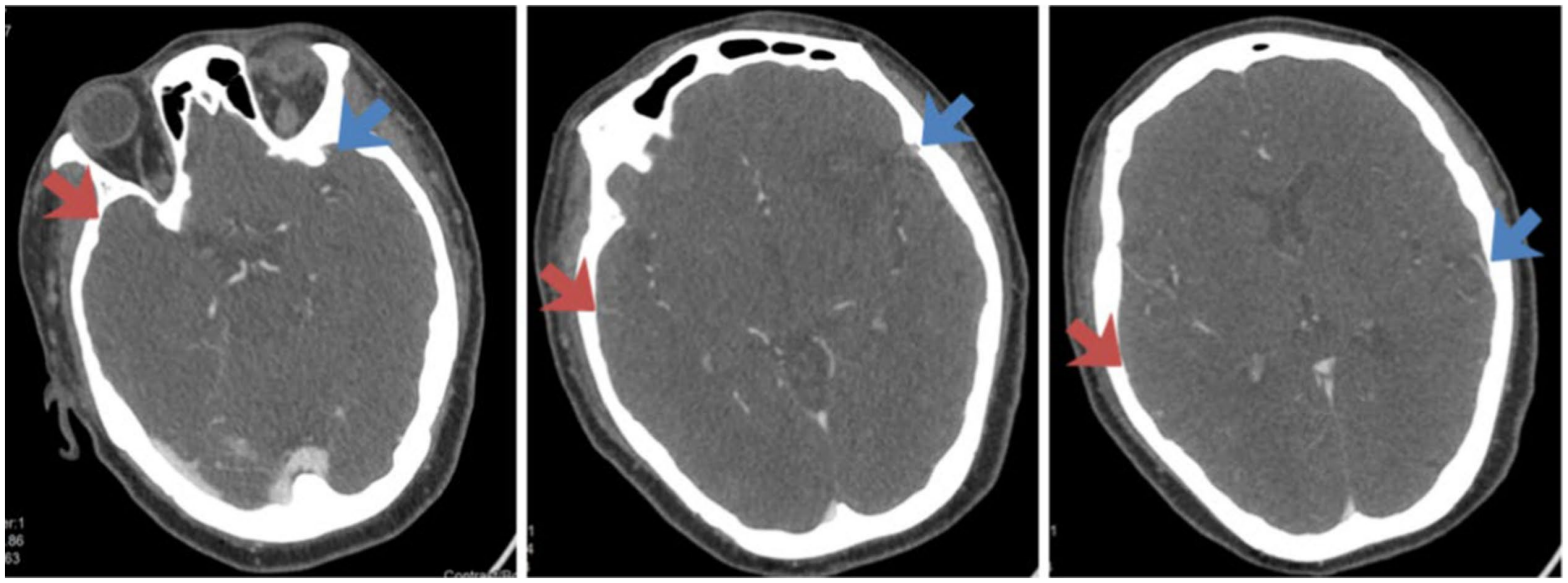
Assessment of venous outflow on the CTA images of Case 1. The side indicated by the red arrow is the affected side, and the side indicated by the blue arrow is the healthy side. From left to right in the figure, the COVES scores are as follows: SPS is 0 point, SMA is 1 point, LA is 0 point, and the total score is 1 point.
